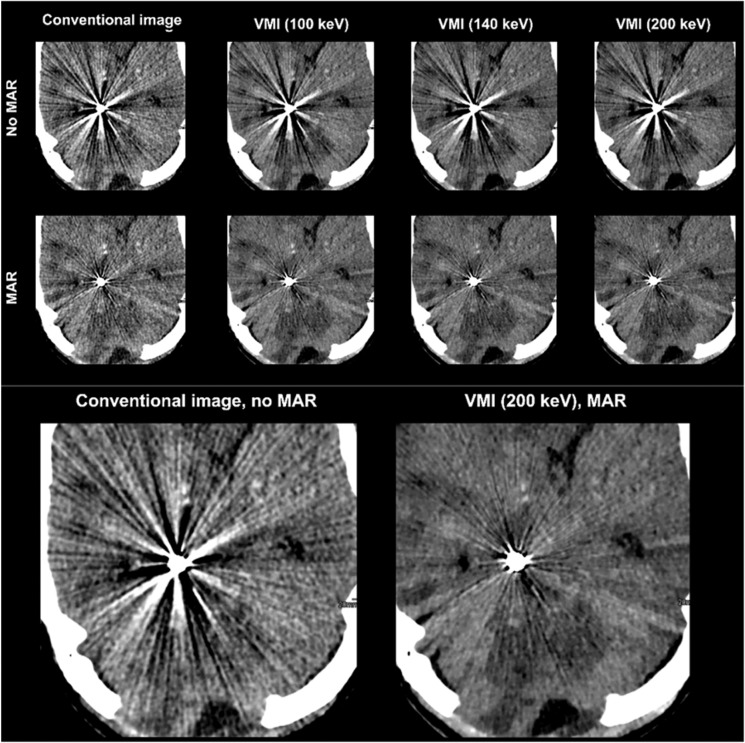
Combination of VMI and MAR reduce even strongest artifacts and relevantly improve assessment of adjacent brain tissue. Conventional CT images (conventional), virtual monoenergetic images (VMI, 100–200 keV), metal artifact reduction algorithms (MAR) and their combination.
